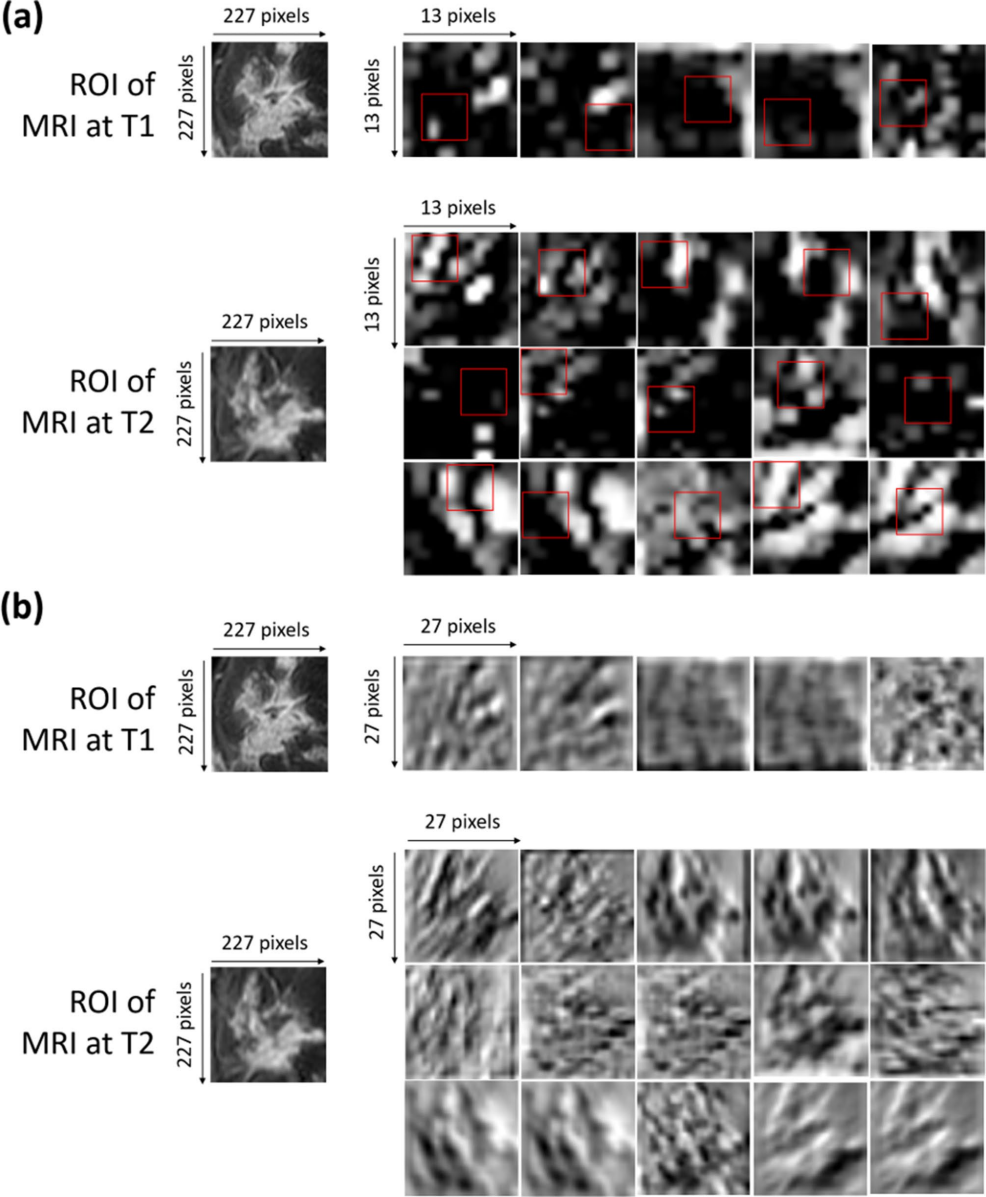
(a) Activation maps of the ROIs of both MRI at T1 and MRI at T2 from which the selected optimal features were extracted. The red squares outline with more precision the area of belonging of such features. Each ROI has dimensions of 227 $$\times$$ 227 pixels. Each activation map has original dimensions of 13 $$\times$$ 13 pixels and has been resized to the dimensions of the corresponding ROIs for a better visualization. (b) Convolutional maps related to the activation maps of the ROIs of both MRI at T1 and MRI at T2 from which the selected optimal features were extracted. Each ROI has dimensions of 227 $$\times$$ 227 pixels. Each convolutional map has original dimensions of 27 $$\times$$ 27 pixels and has been resized to the dimensions of the corresponding ROI for a better visualization.
